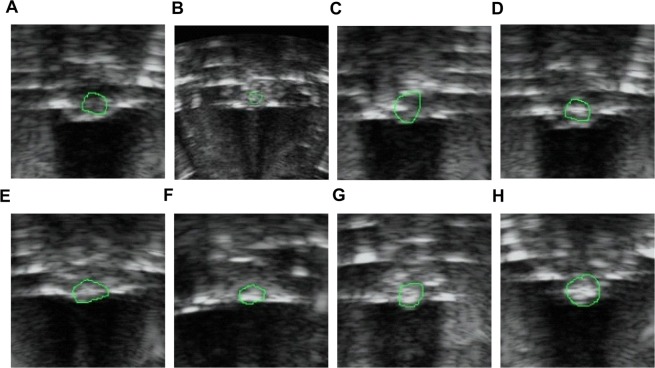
Gray-scale changes of targeted tissues before and after HIFU treatment. (A) Before and after HIFU treatment. (B) HIFU. (C) PLGA+HIFU. (D) PFP/PLGA+HIFU. (E) HMME/PLGA+HIFU. (F) HMME+PFP/PLGA+HIFU. (G) HMME+PFP/PLGA-Ab+HIFU. (H) HMME+PFP/PLGA-SA+HIFU. The tumor showed low echo in ultrasonography before HIFU treatment, and the gray-scale of the tumor target tissue area in all groups changed in varying degrees after the HIFU treatment.
